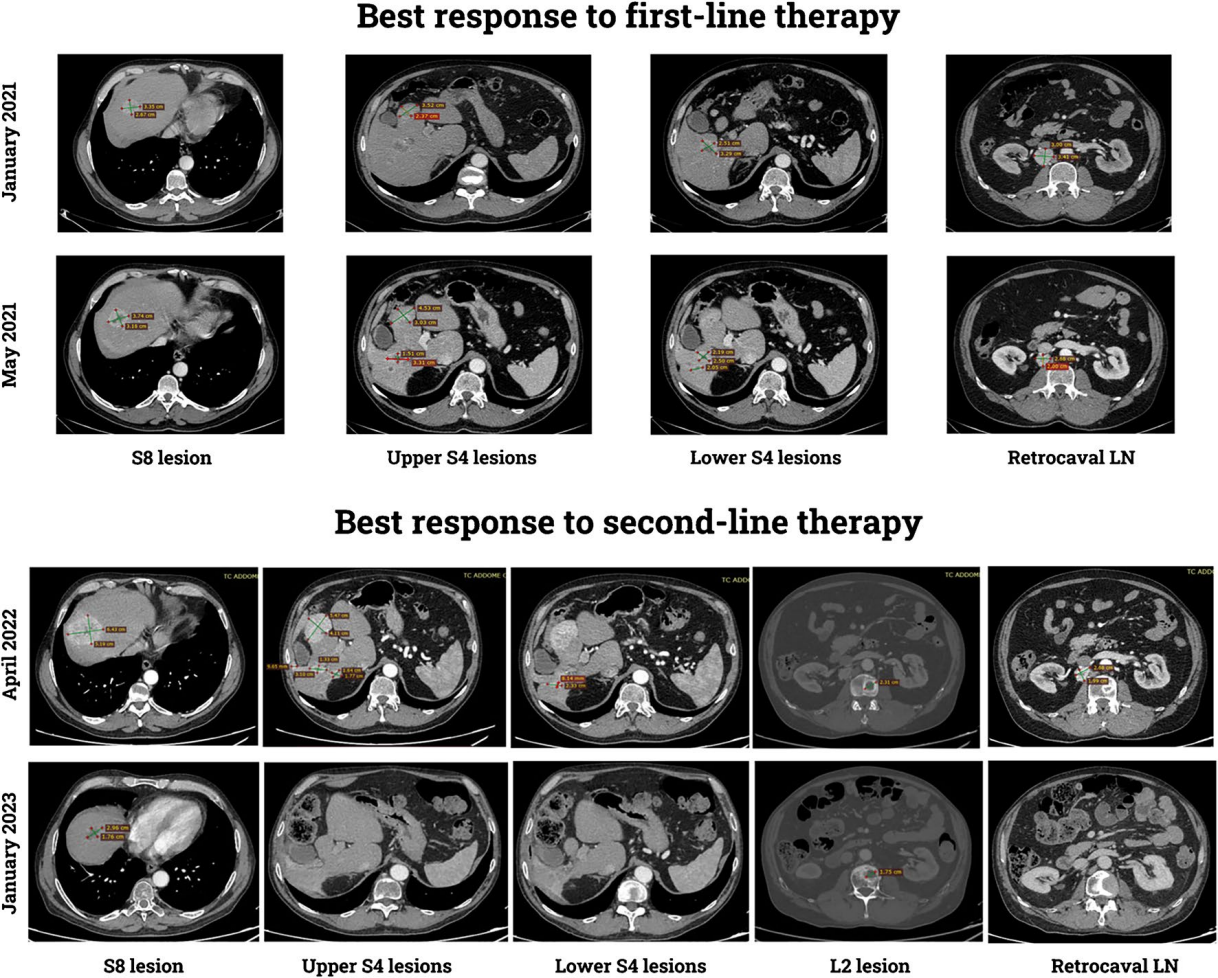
Comparison between the baseline CT scan performed before the start of the first-line therapy with Pembrolizumab and Axitinib (January 2021), at the best response to the first-line therapy (May 2021), at the progression to the first-line therapy (April 2022), and after 9 months of the second-line therapy with Lenvatinib and Everolimus. Little to any tumor shrinkage was observed with Pembrolizumab and Axitinib. Conversely, following Lenvatinib and Everolimus, just a few hepatic lesions were detected, showing a decrease in size and vascularization. Disease stability for the osteolytic lesion in L2 was also recorded. All the images were taken in the arterial phase
